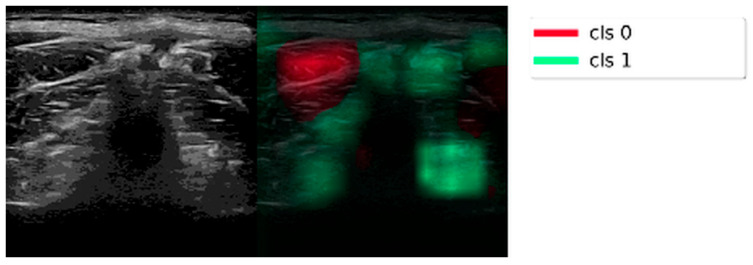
The Sample Output of Grad-CAM. It shows how the model determines which parts of the input are more relevant to the features of class 0 or class 1. In this sample, only the red highlighted part led the model to classify it as class 0. (Note: All four features—Deep Fascia Discontinuity, Deep Fascia Fuzzy Texture, Deep Fascia and Myofascial Adhesion, MHS—are evaluated based on the same ultrasound image, so only one Grad-CAM visualization is shown).
